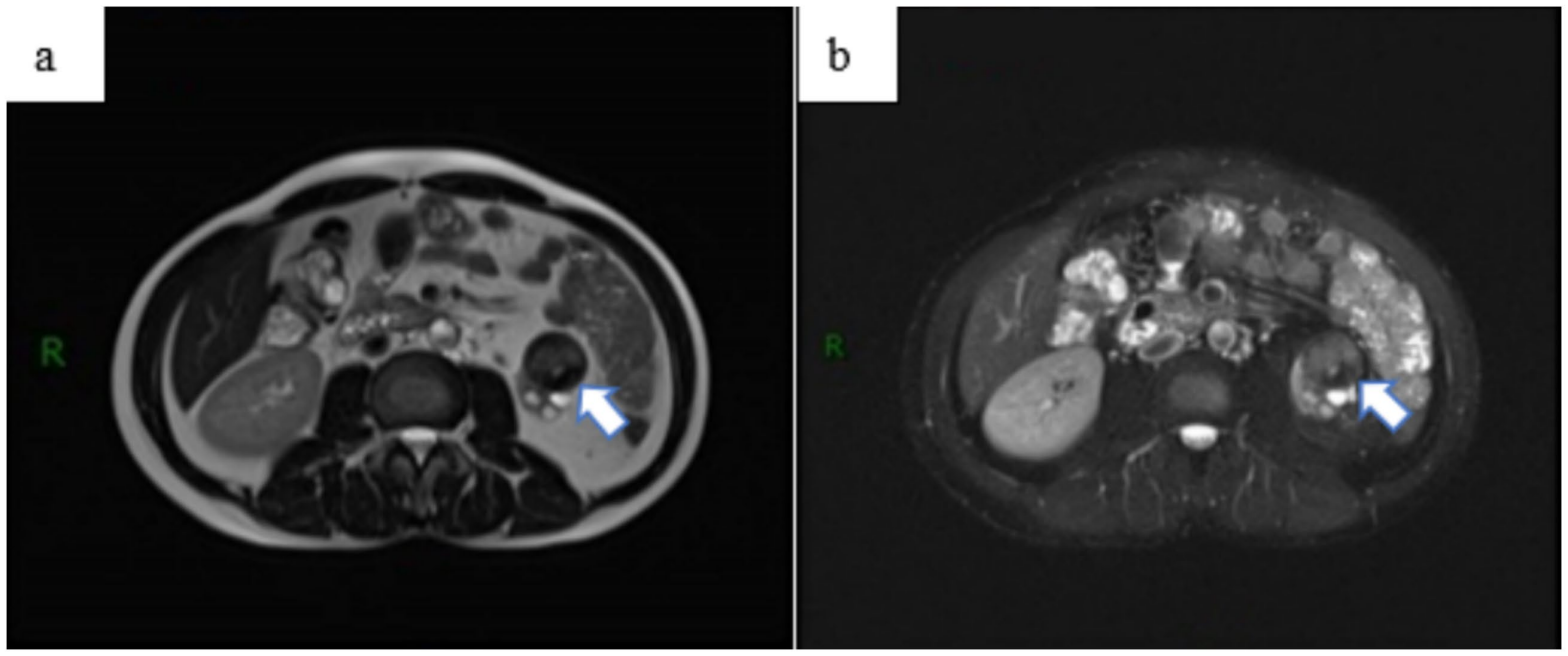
Abdominal MRI examination revealed a space-occupying lesion outside the renal contour of the left kidney, (a) with no significant enhancement observed on the enhanced scan (b). The lesion was classified as a renal tumor, stage T3aN0M0.
